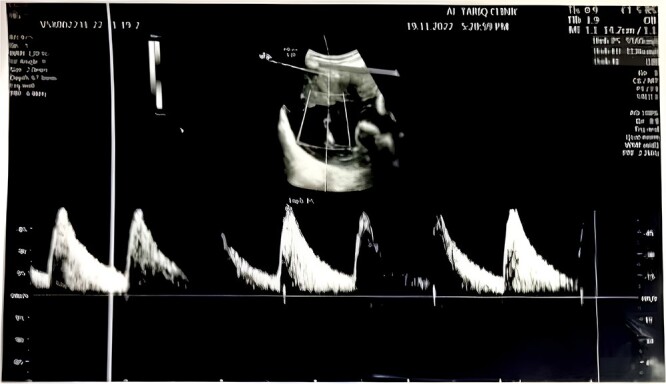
Neonatal Doppler ultrasound showing a single viable, active, and normal-tone female fetus in cephalic presentation and longitudinal lie. Gestational age: 31 weeks. Estimated fetal weight (EFW) = 1950 grams, with no sonographic signs of intrauterine growth restriction (IUGR). Normal amount of amniotic fluid and no gross fetal congenital abnormalities observed. Normal middle cerebral artery blood flow and spectral waveform. Biophysical profile = 8/8.
